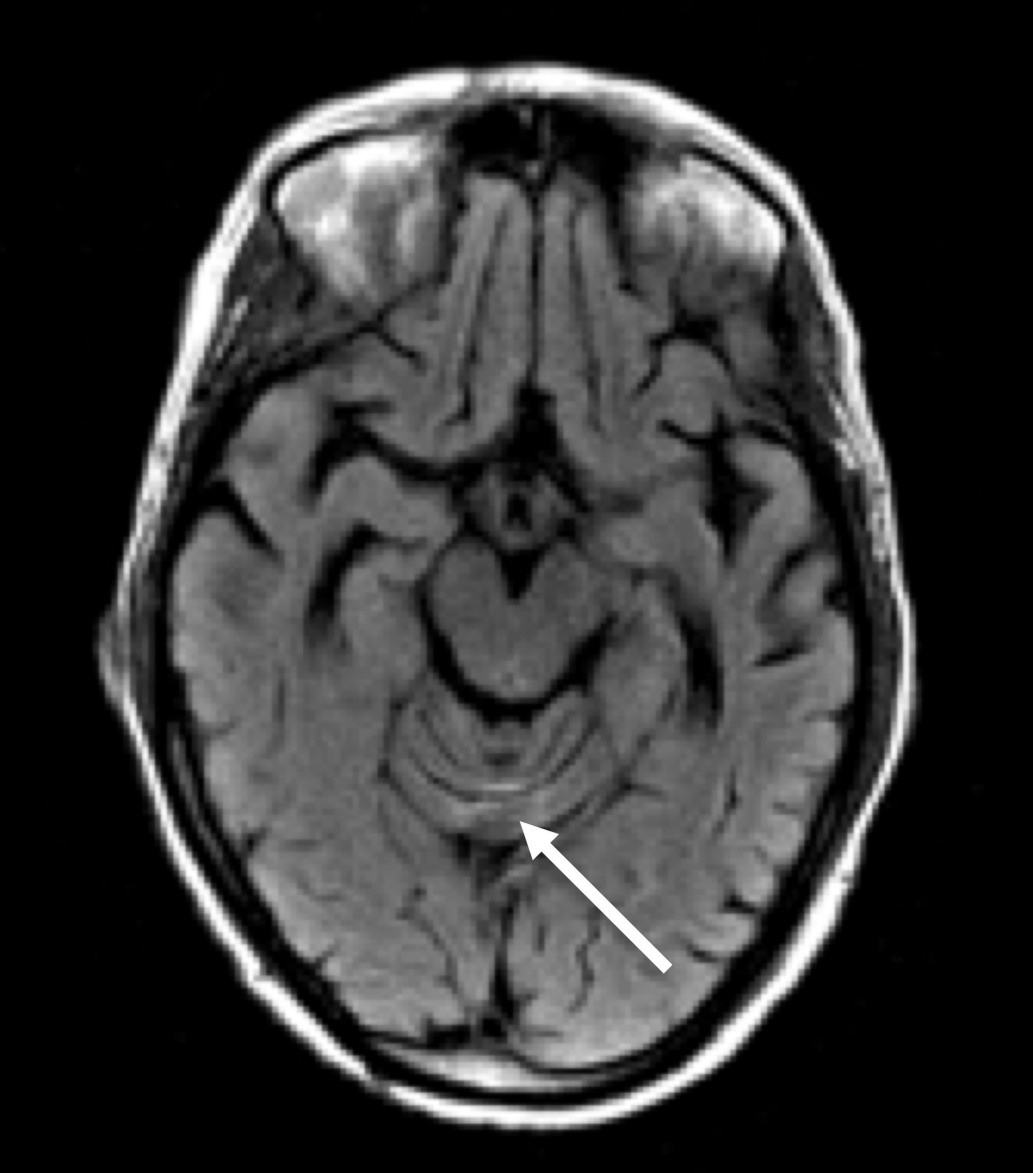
Axial FLAIR MRI of the head Axial FLAIR MRI of the head at the level of the midbrain shows abnormal increased signal in the superior vermis and prominence of vermian folia (arrow)
